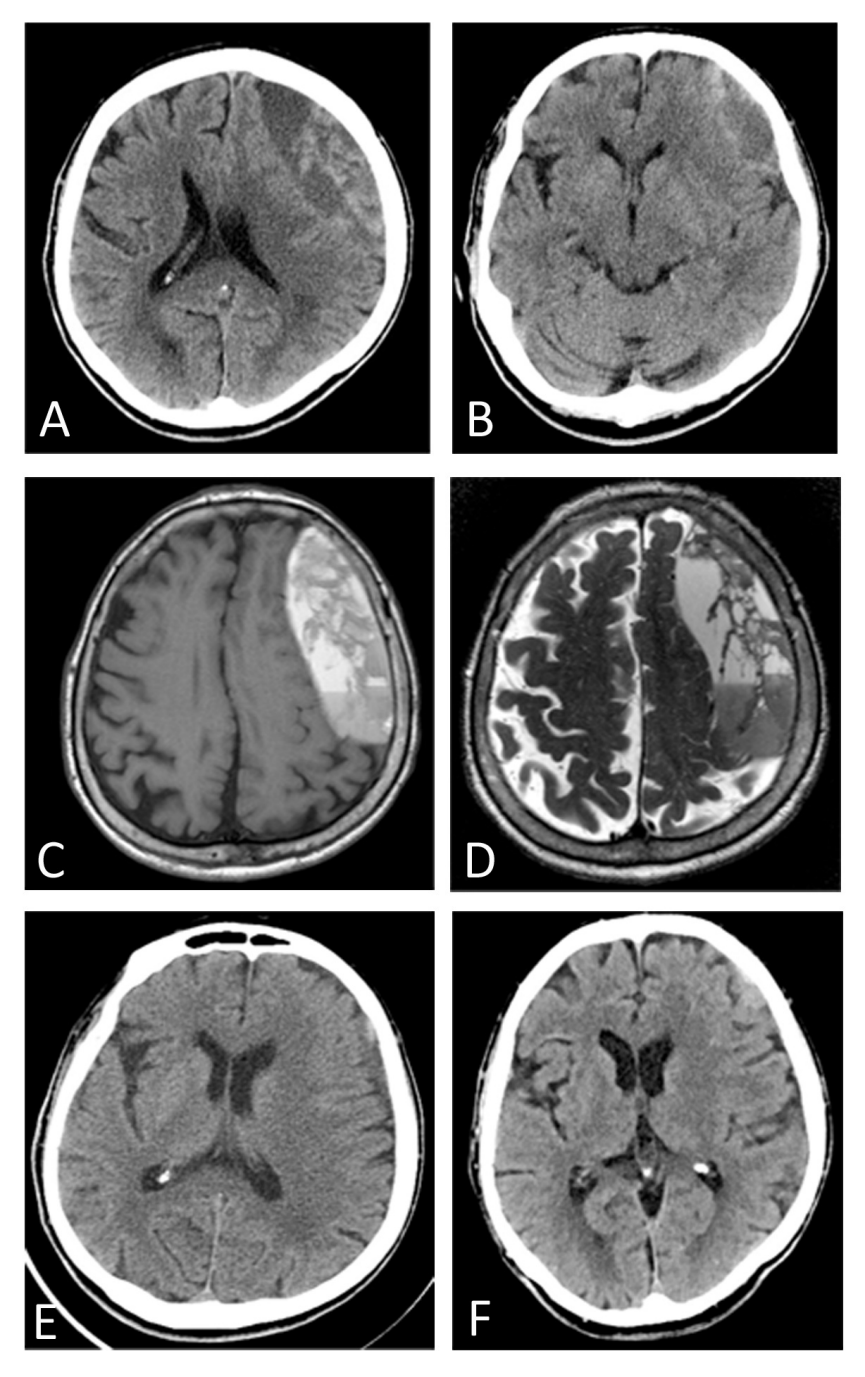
Pre and postoperative imaging of chronic subdural hematoma Preoperative non-contrast CT scan (A,B), T1 weighted (C), and T2-weighted (D) MRI scans demonstrating subacute on early chronic left convexity subdural hematoma causing local mass effect and left to right midline shift. Postoperative non-contrast CT scans at one month (E) and two months (F) postoperative showing signficant improvement in evacuation of hematoma and resolution of midline shift and local mass effect.
